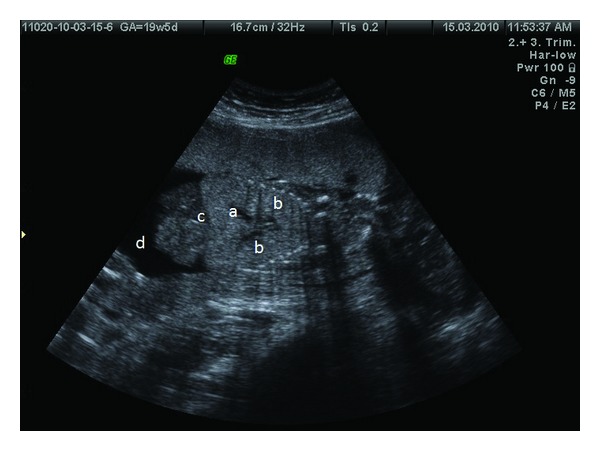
Antenatal sonography of case 1 showing dilated main bronchi (a), large hyperechoic lungs (b), inverted diaphragm (c), and ascites (d).
